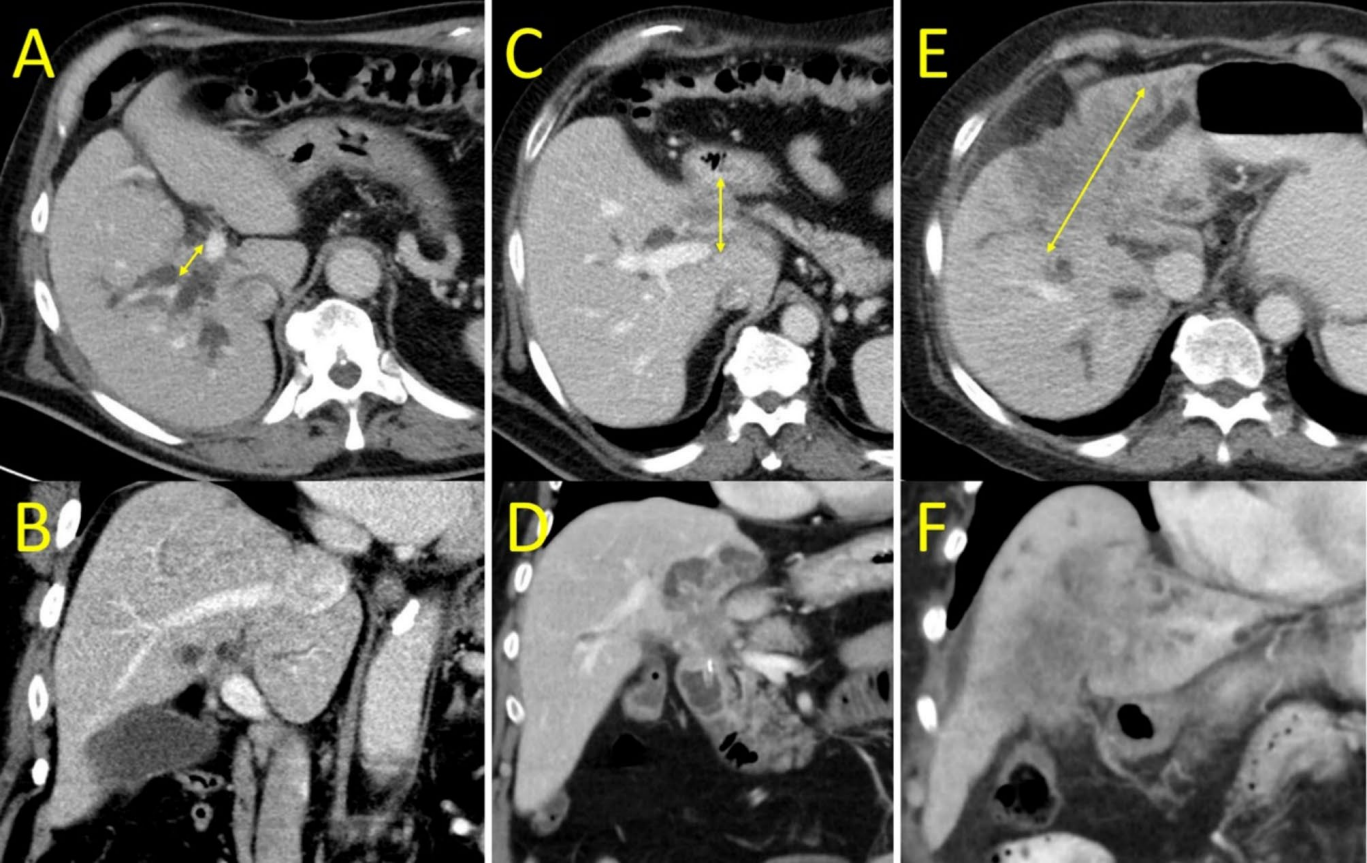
Contrast-enhanced CT in the portal phase in the axial (A, C, E) and coronal planes (B, D, F). Figure A and B show infiltrative cholangiocarcinoma, Figure C and D show a mass of cholangiocarcinoma of the size 2 to 5 cm, and Figure E and F show a mass of cholangiocarcinoma larger than 5 cm. The arrows demonstrate the method of measuring the maximum diameter of each type of cholangiocarcinoma on CT scans in axial planes.
